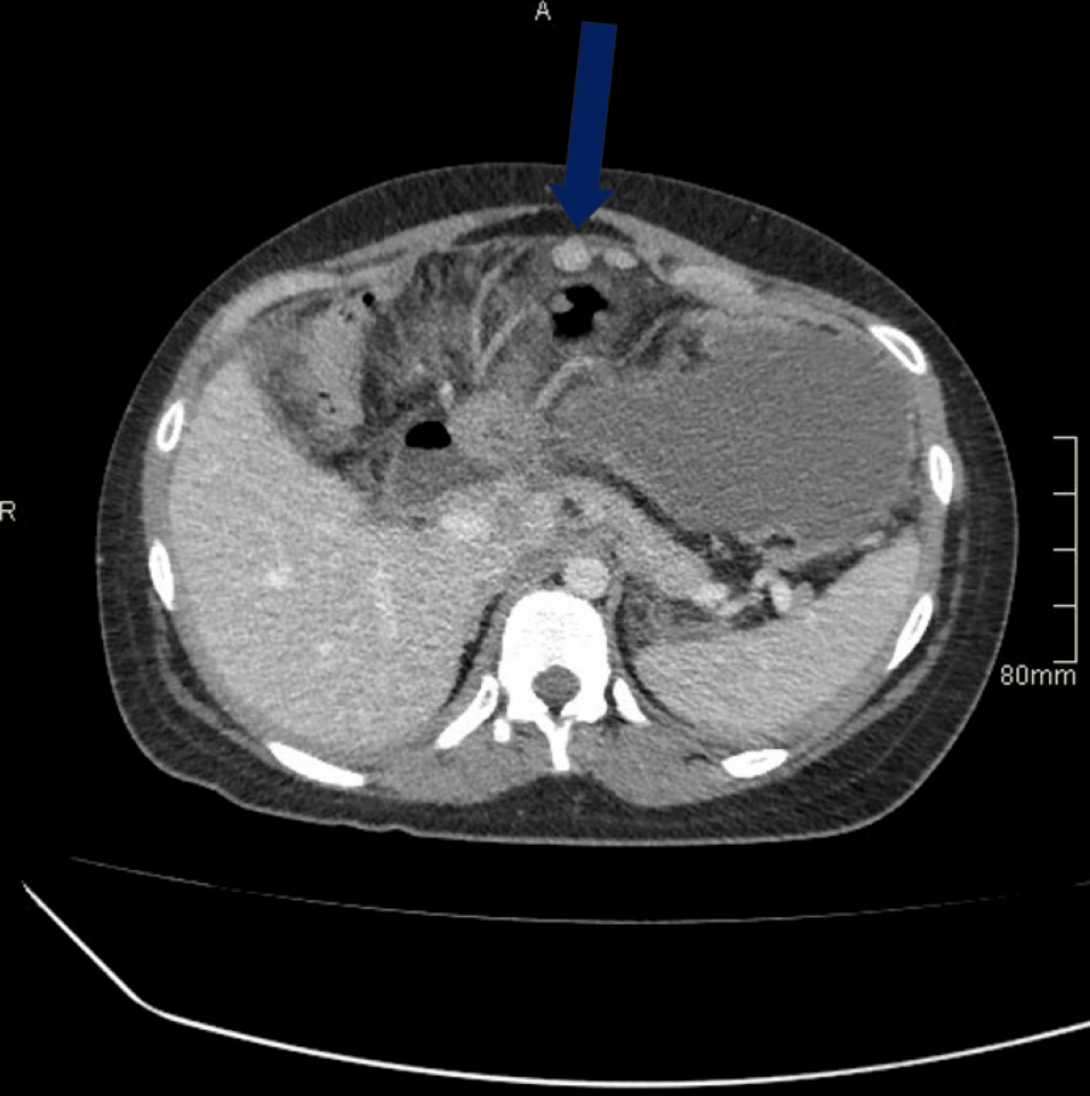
CT abdomen on initial presentation. The arrow points to peri-gastric lymph nodes, which show peritoneal nodularity and ascites, consistent with peritoneal tuberculosis mimicking malignancy.
